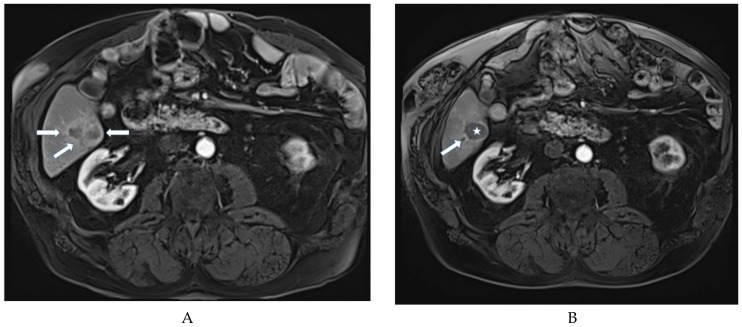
(A) Liver MRI (arterial phase) showing a 4-cm HCC (arrows) in segment VI in a 71-year-old cirrhotic patient. (B) MRI (arterial phase) performed three months after a super-selective DEB-TACE session (using 10 mg idarubicin loaded in 75-µm TANDEM beads administered through the segment VI artery) showing complete response (star) upon mRECIST, with dilatation of the segmental bile duct (arrow).
